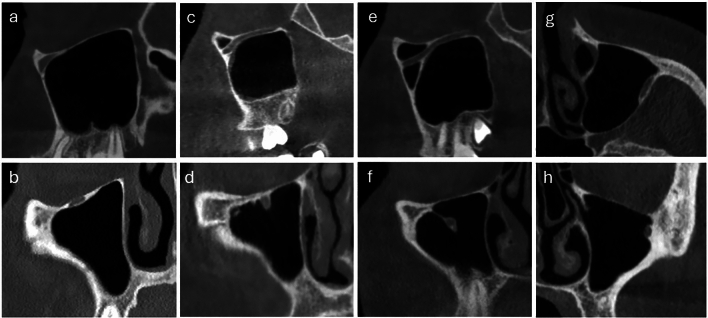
Sagittal and coronal CBCT images of, a-b Type I infraorbital canal (IOC), c-d Type II IOC, e-f Type III IOC on the right side, g-h axial and coronal CBCT image of Type IV IOC on the left side, and h IOC dehiscence
